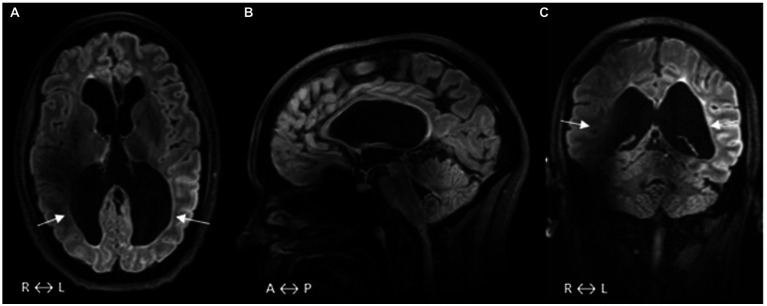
(A–C) FLAIR-MRI acquired at 7 T. A–C show a general loss of WM with secondary ventricular dilatation (A), small areas of gliosis in the periventricular WM, and size reduction of thalami (A). The WM volume loss is most pronounced in the parietal regions with the expected affection of each of the ORs (arrows A and C). Midsagittal slice (B) shows that the corpus callosum is extremely thin, especially its posterior part. Magnetic field inhomogeneities, a common problem at 7 T, result in signal inhomogeneity/loss in the vicinity of tissue/air interfaces such as temporal bones and paranasal sinuses (A–C). WM, white matter; FLAIR, fluid-attenuated inversion recovery; OR, optic radiation; R, right; L, left; A, anterior; and P, posterior.
